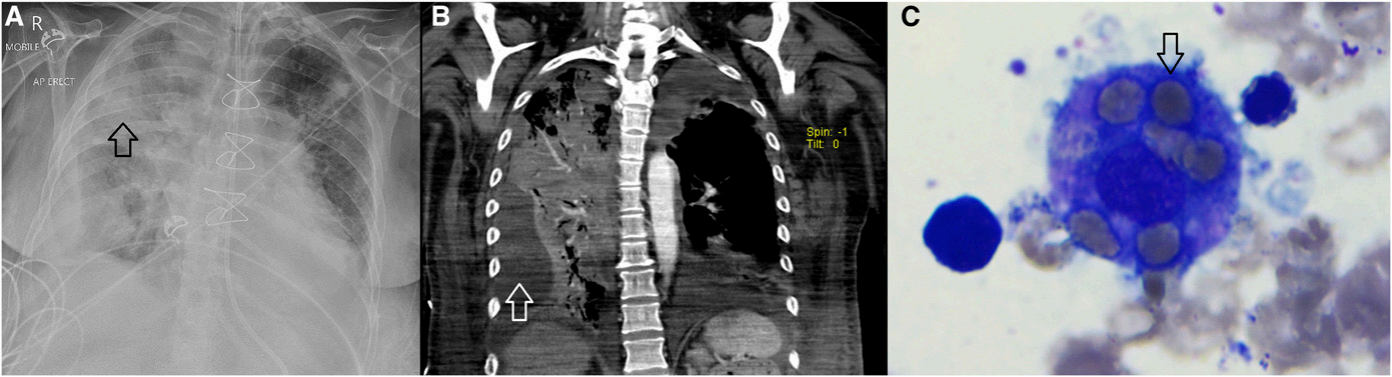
(A) Anteroposterior chest X-ray showing extensive consolidation (arrow). (B) Coronal computerized tomography images confirming extensive pneumonia and revealing a large right-sided pleural effusion (arrow). (C) A histiocyte with arrow pointing to mature erythrocyte phagocytosed within the cytoplasm. At least six mature erythrocytes are seen within this cell. This figure appears in color at www.ajtmh.org.
